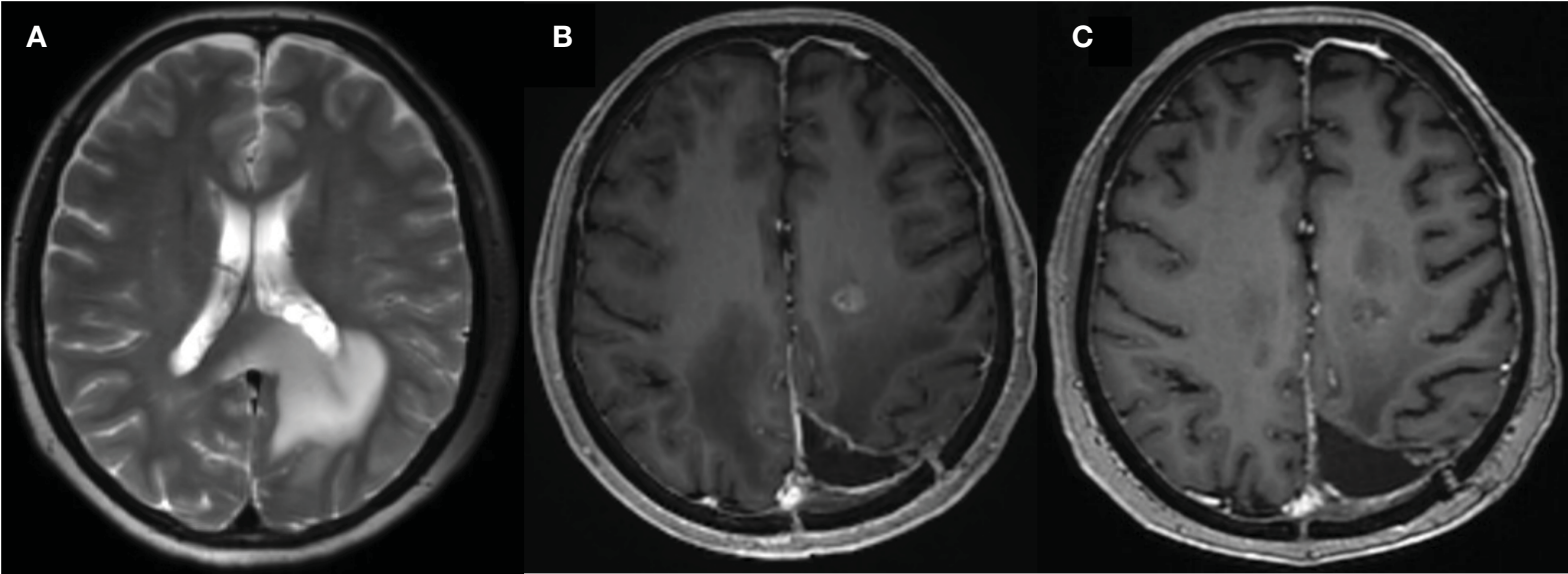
A 59-year-old female with glioma of the left parieto-occipital lobe, treated with surgery and radiation therapy. (A) MR image before surgery; (B) 10 months after surgery and completion of radiation therapy showed enhancing lesion; (C) follow-up MR showed resolution of the lesion.
